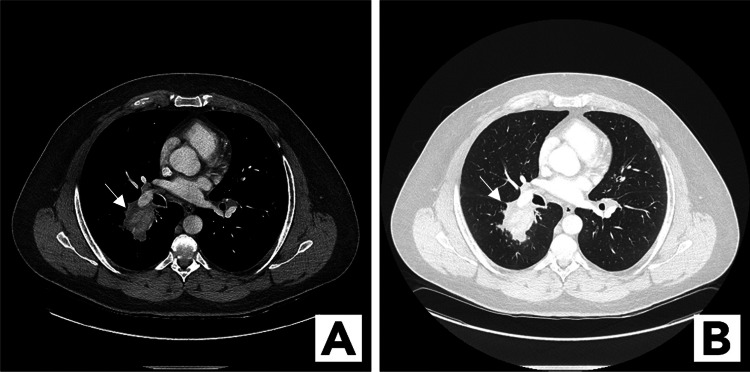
Hilar mass Mediastinal window (A) and lung window (B) CT revealed a solitary 57 x 48-mm hilar mass (arrows) associated with hilar adenopathy CT: computed tomography
